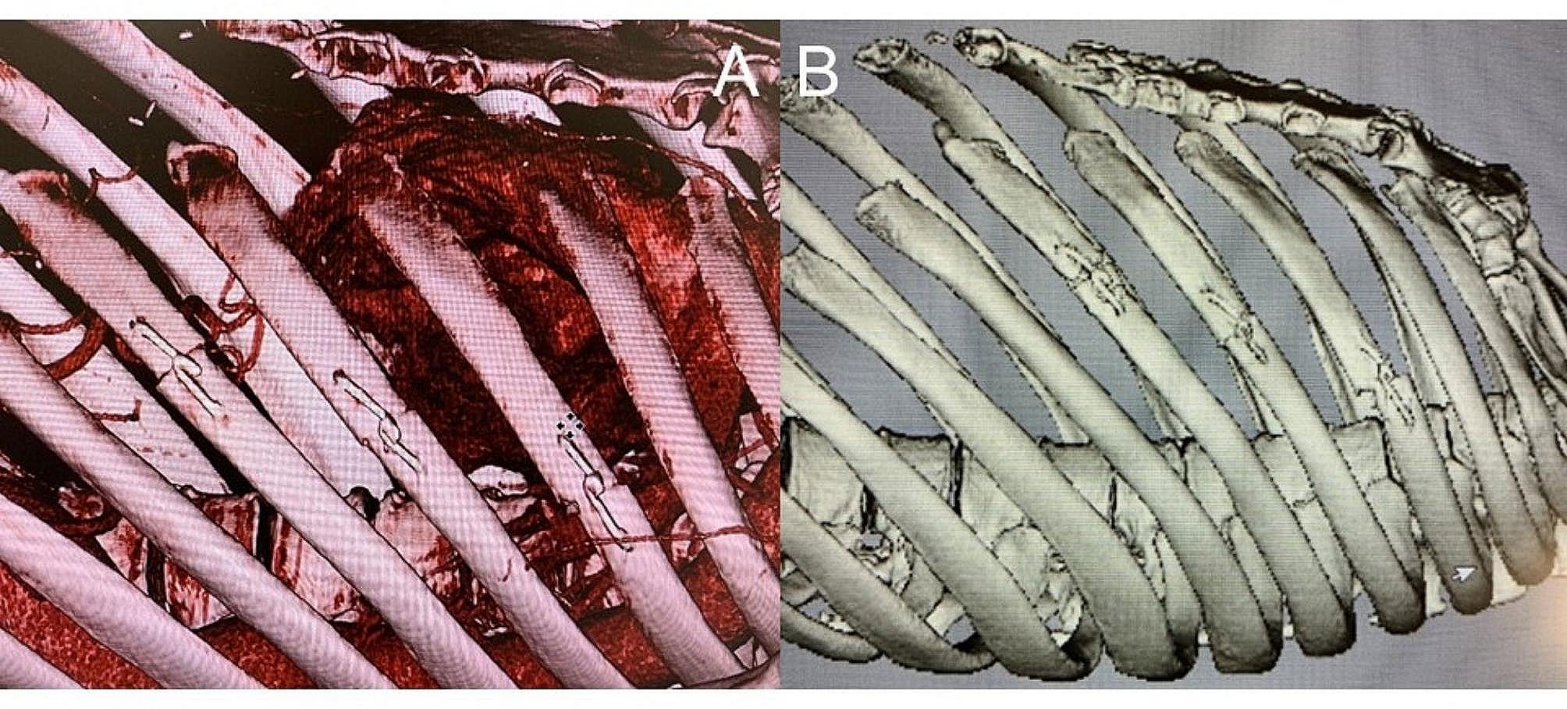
Computed tomography before discharge shows the fixed ribs (A) Computed tomography at eight weeks after surgery demonstrates fixed ribs. One incised rib is vertically displaced (B)
